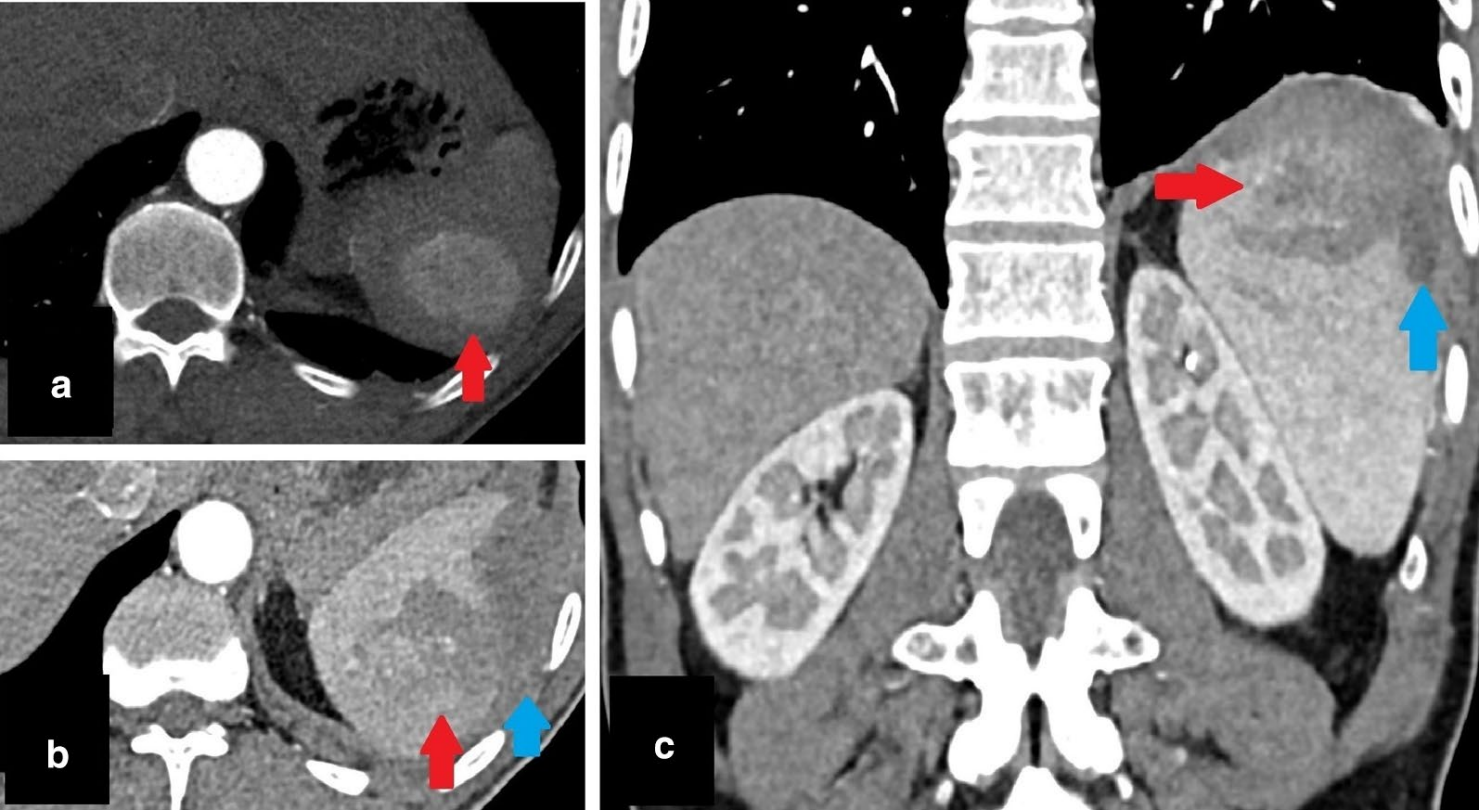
Delay in diagnosis with a life-threatening condition. A 47-year-old male patient presented with complaints of chest pain and abdominal discomfort. History and clinical examination suggested cardiovascular etiology as a possible underlying cause. Coronary CTA was ordered to evaluate the presence of coronary artery disease. The coronary arteries were reported as normal. However, a well-defined splenic hypervascular lesion was missed due to the “edge effect”. The lesion was seen only on the last few slices of “the CT images with a large field of view” (red arrow, a). One week later, the patient was admitted to the ER with severe left upper quadrant pain. Abdominal CECT revealed a ruptured lesion (red arrows, B, c) and subcapsular splenic hematoma (blue arrows, b, c) with active extravasation (not shown)
